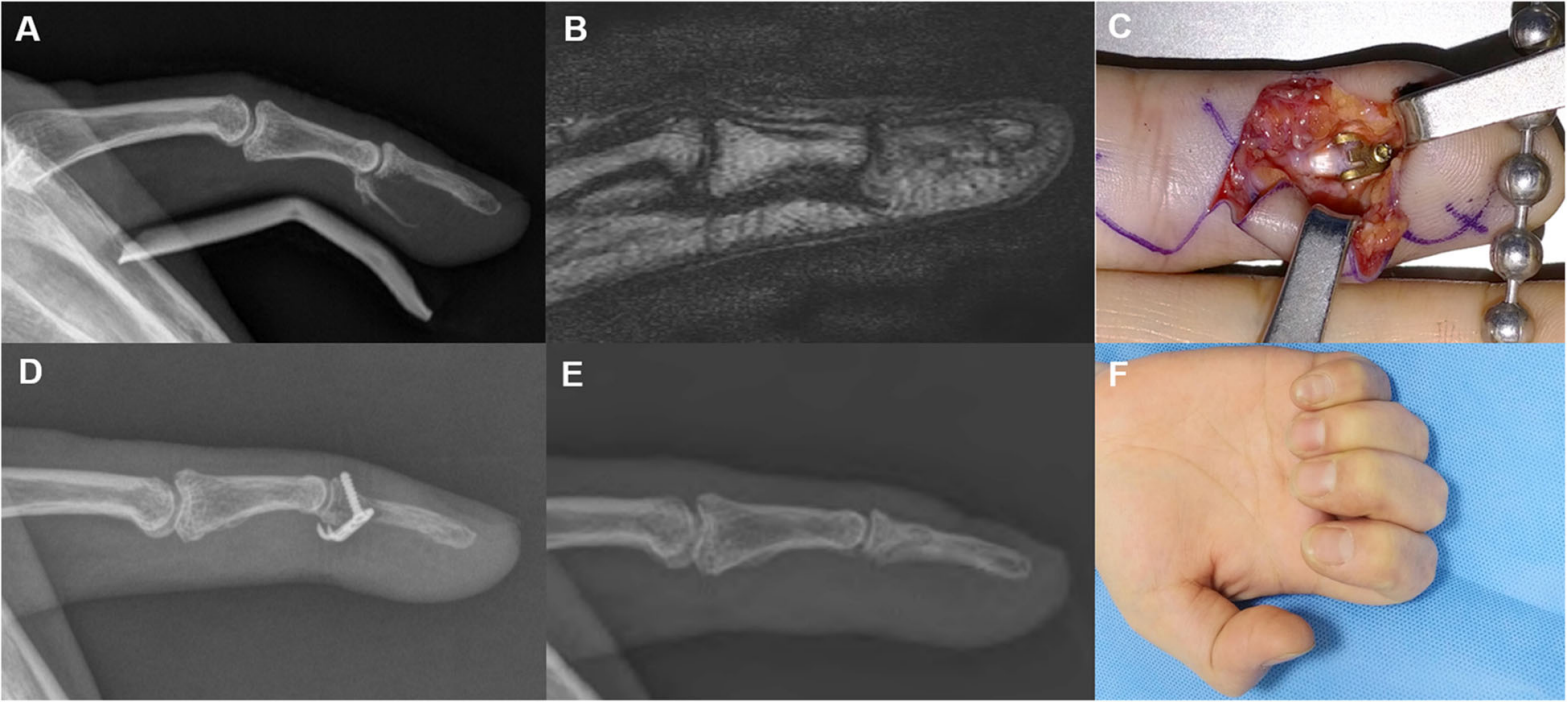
Flexor digitorum profundus (FDP) avulsion fracture (a, b). A mini-hook plate captured the avulsed fragment with FDP (c, d). The patient underwent plate removal and plain radiography 6 months post-operation reveals that the osseous fragment was well united and that there was no joint subluxation. The total range of DIP joint motion was 80%, and the pinching strength was 90% compared to the values of the same contralateral joint (e, f)
